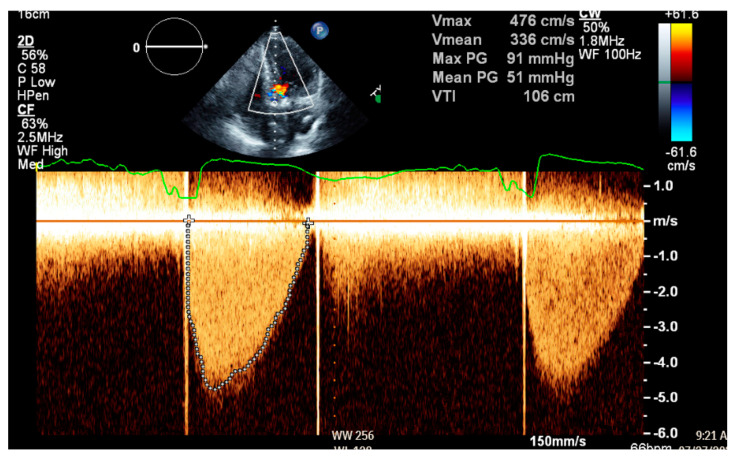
Transthoracic echocardiography Doppler prior to ultraslow low-dose alteplase infusion showing a mean aortic transvalvular gradient of 51 mmHg and aortic valve peak velocity of 494 cm/s, consistent with severe aortic stenosis.
